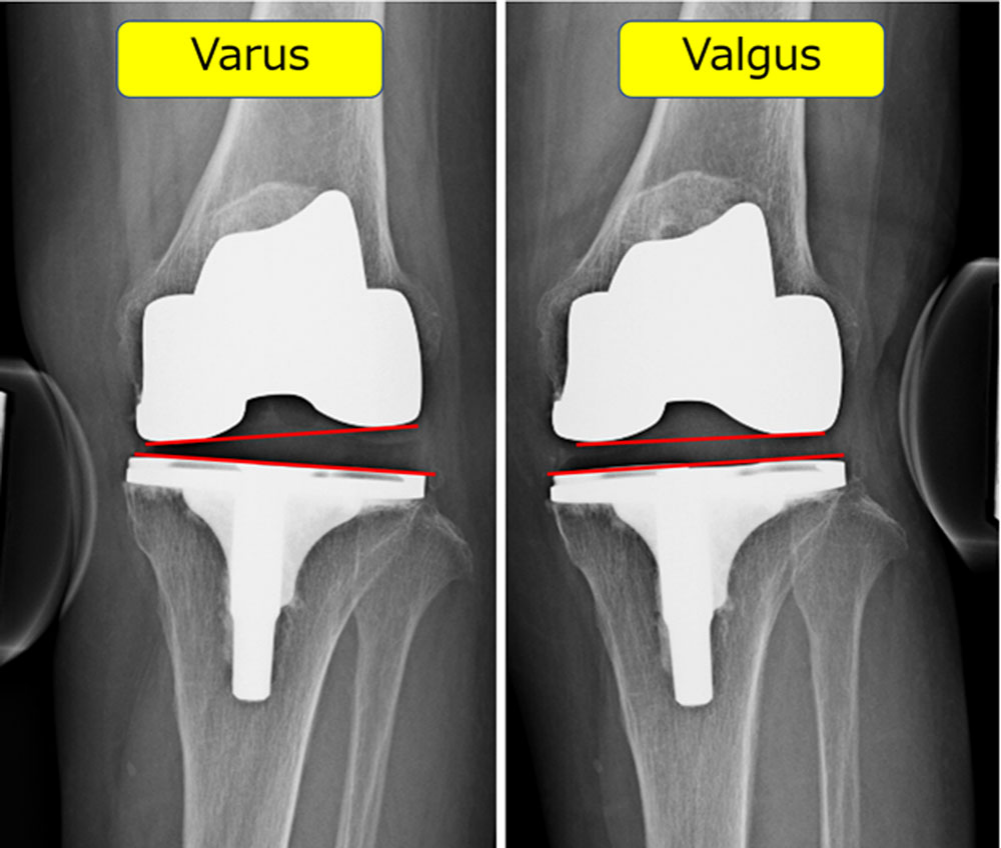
Stress radiographs after total knee arthroplasty. Varus and valgus stress radiographs were used to evaluate the stability of the knee during extension. The stress angle measured was deemed to be positive if the lateral gap was greater than the medial gap. Three degrees were subtracted from the angles measured.
